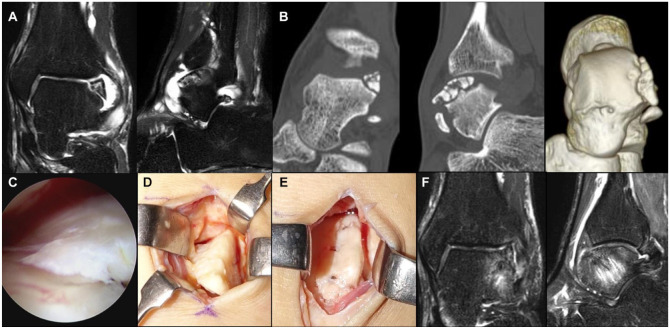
Case 1. (A) Pre-operative magnetic resonance imaging (MRI). (B) Pre-operative computed tomography (CT) images. (C) Arthroscopic findings. The smooth surface of the osteochondral fragment is observed. (D) Macroscopic findings. (E) After fixation using bio-absorbable pins. (F) Post-operative MRI at 1 year after surgery.
